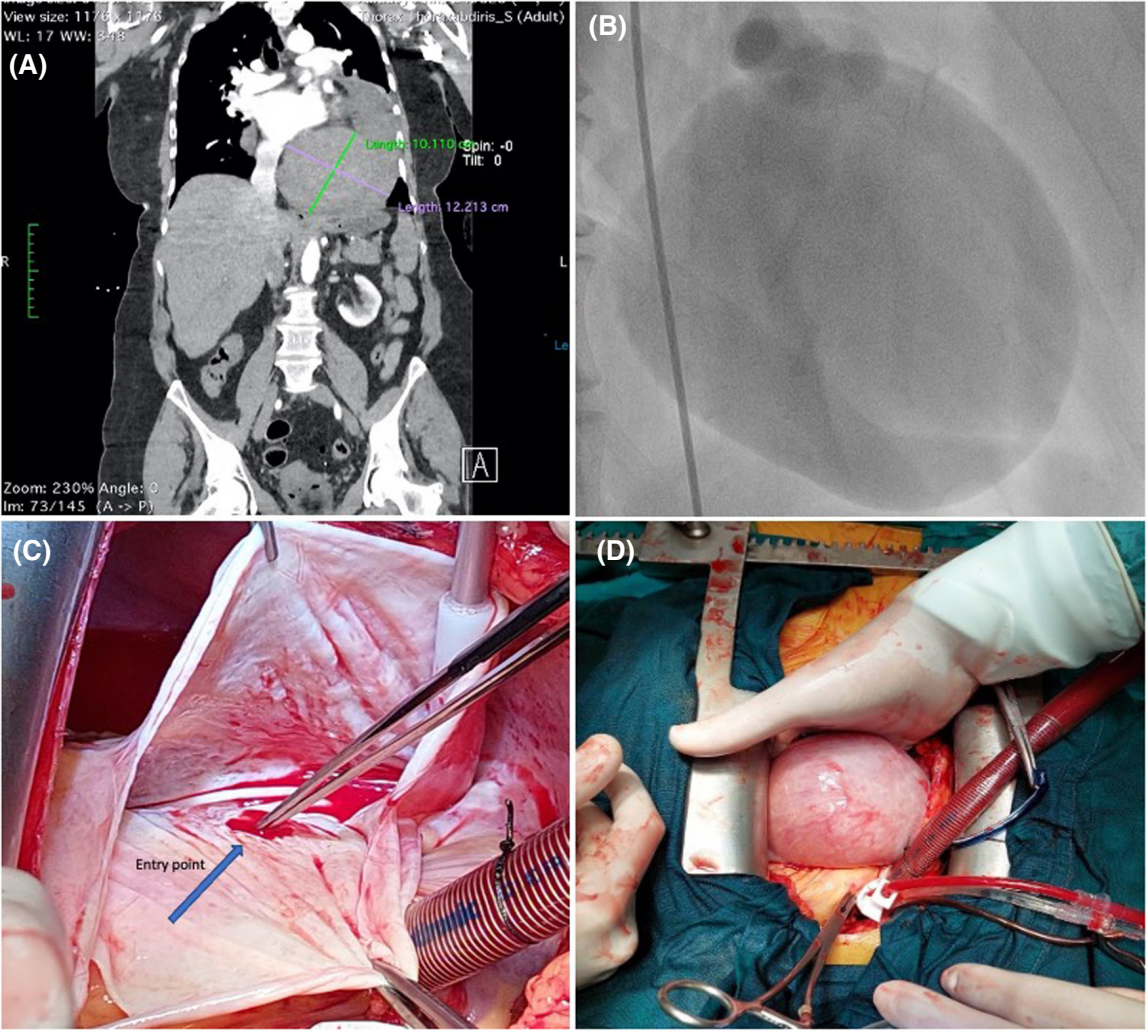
(A) Preoperative CT. The aneurysm measured 10 × 12 cm. (B) Coronary angiography. (C) The aneurysmal sac was opened, and the inflow (entry) point is visible. (D) Intraoperative image of the aneurysm (from patient's head).
